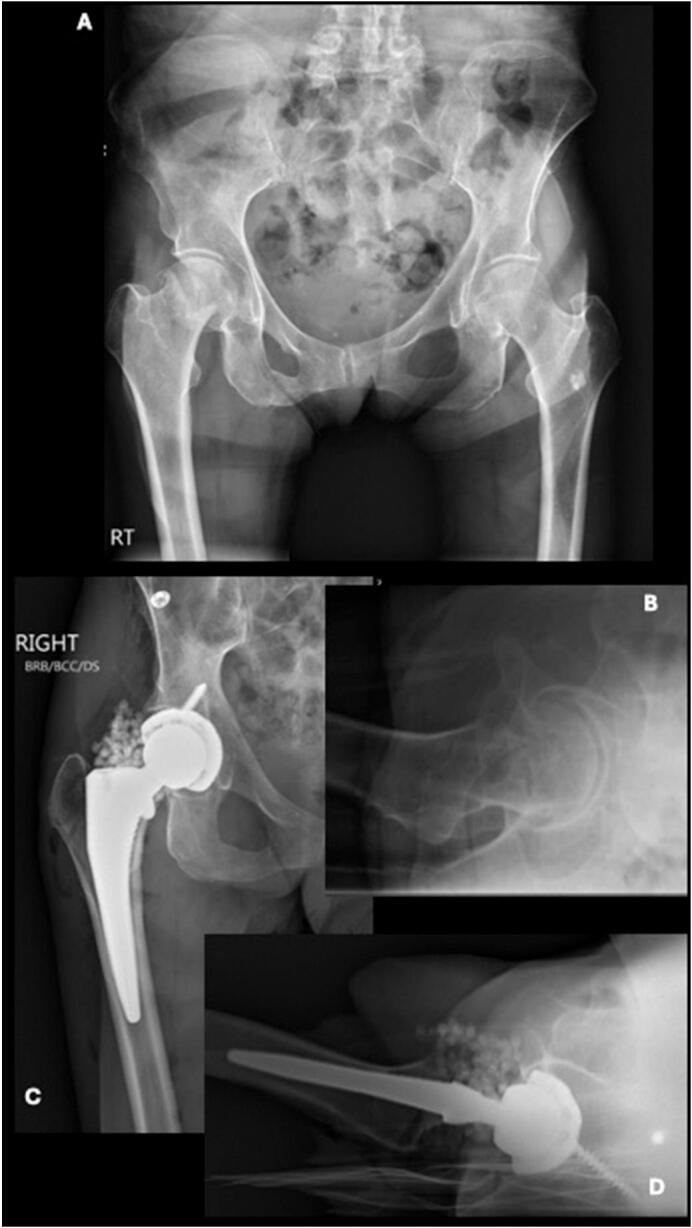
AP (A) and lateral (B) radiographic images of right hip showing displaced right subcapital femoral neck fracture. AP (C) and lateral (D) radiographic images of right hip status post right total hip arthroplasty with placement of antibiotic beads. The beads were used for infection prophylaxis in a high-risk patient, as is standard practice at our institution, particularly in the context of revision surgery when the risk of infection is elevated.
